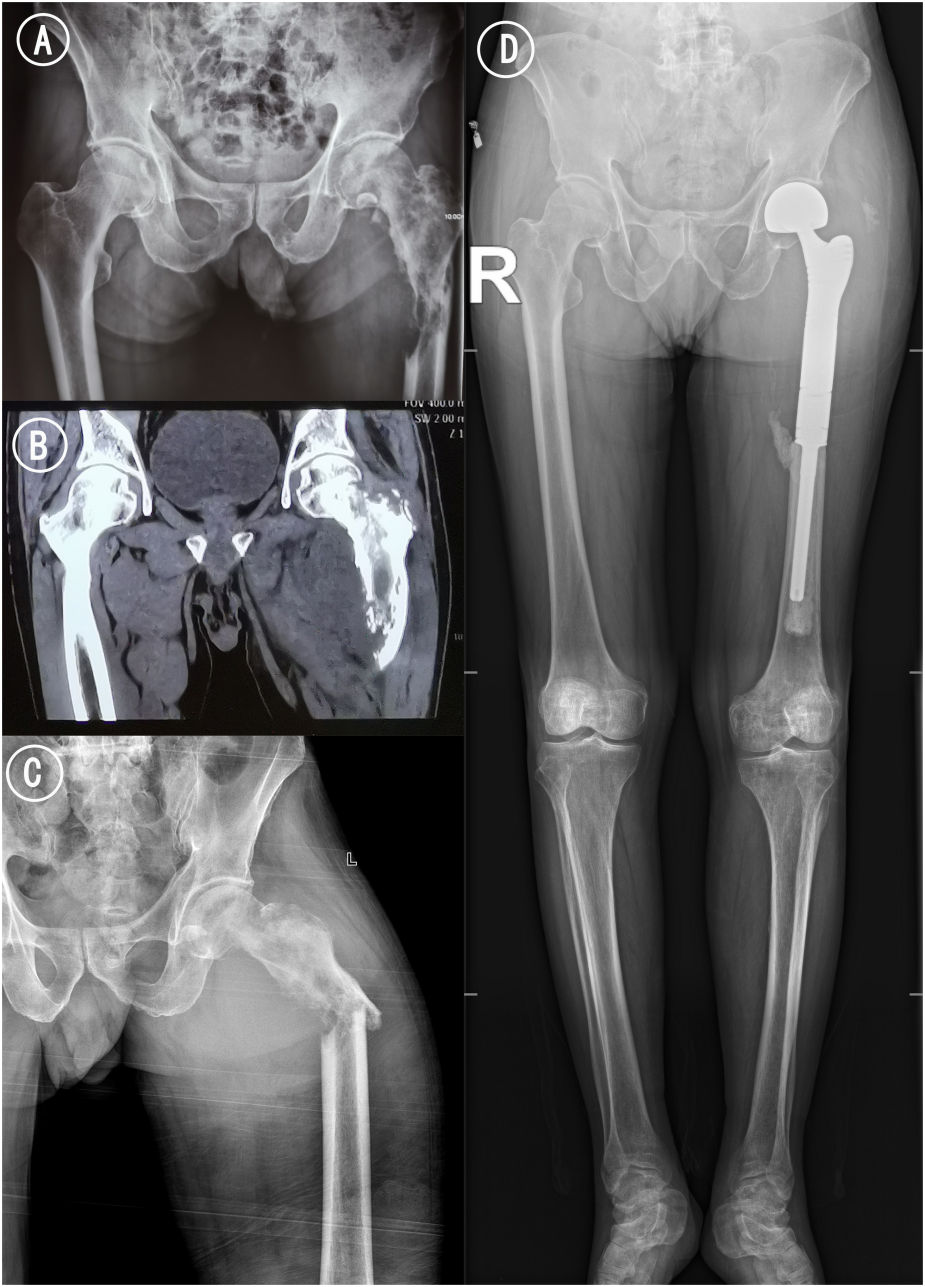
Radiograph and CT of femur. (A) Pelvic ap before pathological fracture. (B) Coronal CT scan of bilateral hip. Osteolytic bone destruction was observed in the upper left femur. (C) Left hip ap x-ray examination after pathological fracture. The femoral cortex was discontinuous, angulated and deformed, and the soft tissue was swollen. (D) Postoperative X-ray of the long-leg radiographs 15 months after treatments: the femoral prosthesis was well in place without loosening and abnormal radiolucent lines.
